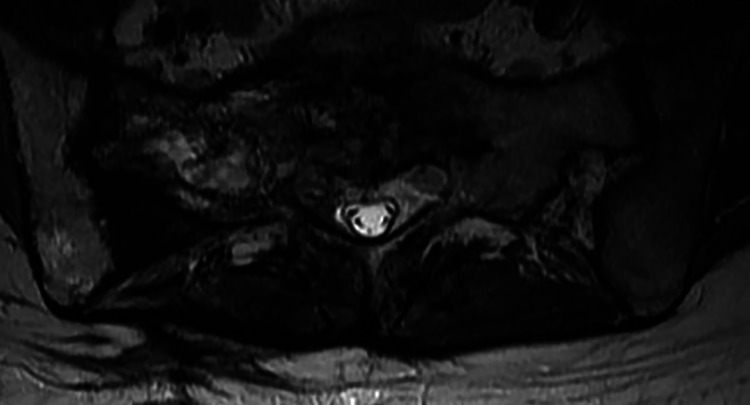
Axial T2-weighted fast recovery fast spin-echo (FRFSE) MRI of the pelvis Axial T2-weighted fast recovery fast spin-echo (FRFSE) MRI of the pelvis demonstrating an ill-defined lesion representing Ewing's sarcoma within the sacrum (red arrows). The lesion is eccentric to the right involving a large portion of the right sacral ala interspersed with fat, which is compatible with areas of bone infarction. The site of the open surgical biopsy within the right posterior lumbar paraspinal and subcutaneous tissues (blue arrows) is also the site of the posterior sacroiliac joint ligament.
